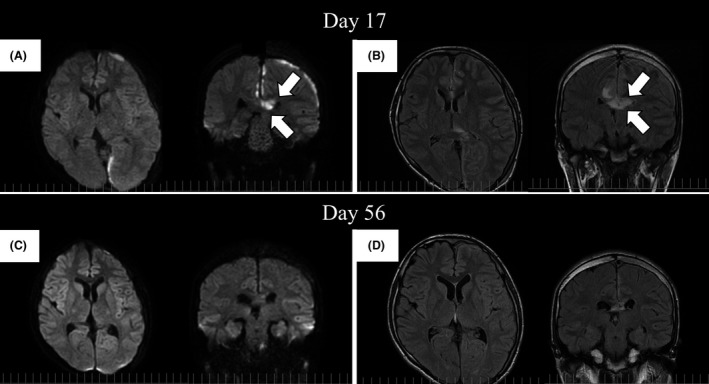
Follow‐up magnetic resonance imaging studies performed on days 17 and 56. (A) The subdural abscess was remarkably diminished in diffusion‐weighted image (DWI) sequences. However, a high‐intensity area appeared in the corpus callosum (arrows) on day 17. (B) In fluid‐attenuated inversion recovery (FLAIR) sequences, the corpus callosum also exhibited high intensity (arrows), and its involvement in the infection was suspected. (C and D) The corpus callosum lesion remarkably improved in both DWI and FLAIR sequences on day 56.
